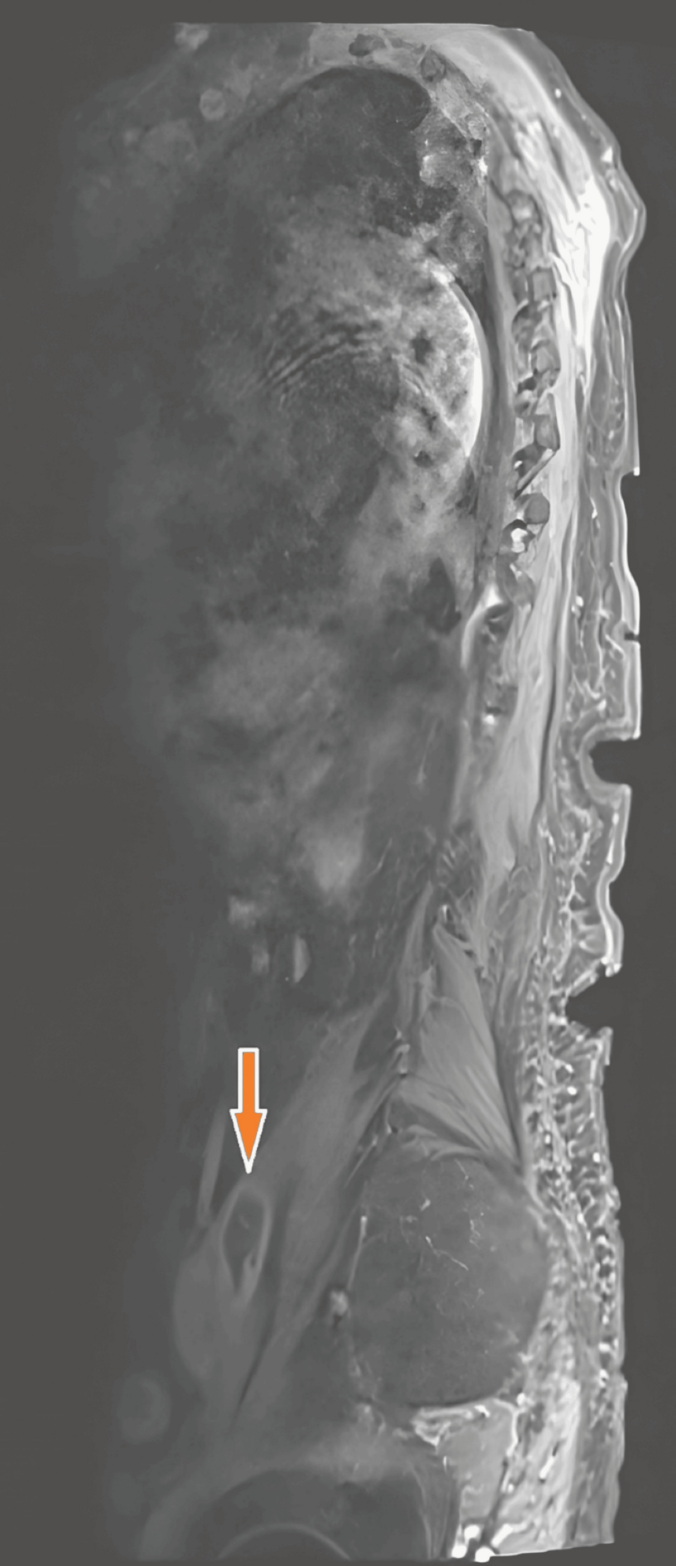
Sagittal MRI of the lumbar spine demonstrating a hyperintense fluid collection along the left psoas muscle, consistent with a psoas abscess. The orange arrow highlights the abscess cavity.
